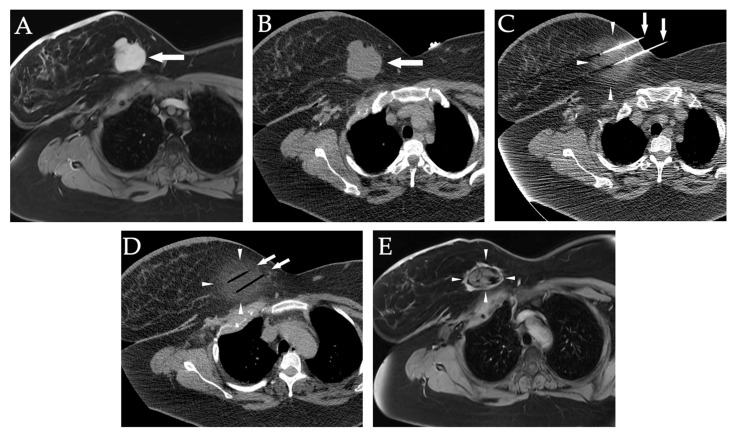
Right breast sarcoma treated with CT-guided cryoablation. (A) Axial gadolinium-enhanced T1-weighted, fat-suppressed MR image shows a lobulated right breast sarcoma mass (white arrow). (B) Axial pre-cryoablation, non-enhanced CT image shows target lobulated right breast lesion (white arrow). (C) Axial non-enhanced CT image during cryoablation shows two cryoprobes (white arrows) traversing the target lesion (white arrowheads). The lesion is less visible having become nearly iso-dense to fat due to freezing. (D) Axial non-enhanced CT image at the conclusion of cryoablation shows the two cryoprobe-ghost trajectories (white arrows) after the probes were removed (white arrowheads). (E) Axial gadolinium-enhanced T1-weighted, fat-suppressed MR image 2-years after cryoablation, shows a shrinking, non-enhancing, necrotic lesion surrounded by a thin rim of fibrous tissue (arrowheads).
